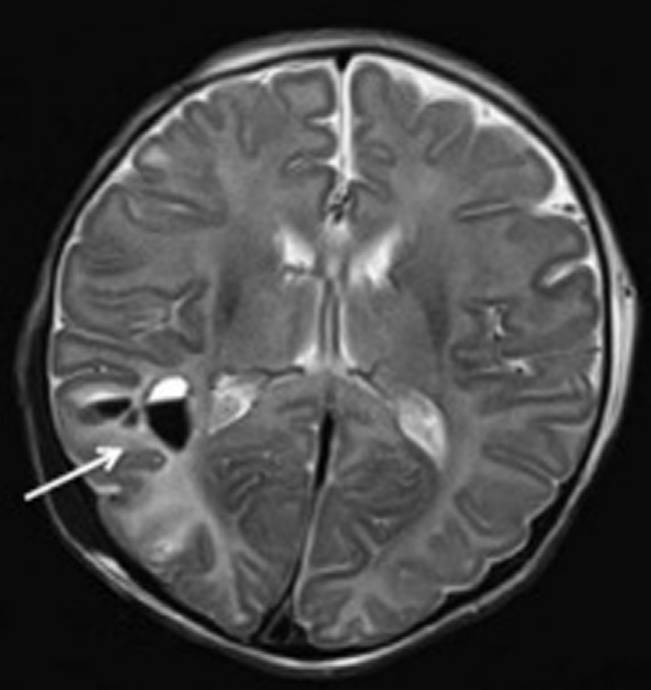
Patterns of enhancement on T2 weighted MRI classified as severe demonstrating right intraparenchymal hematoma (arrow) with surrounding hyperintensity. Figure is reproduced with permission (76).
